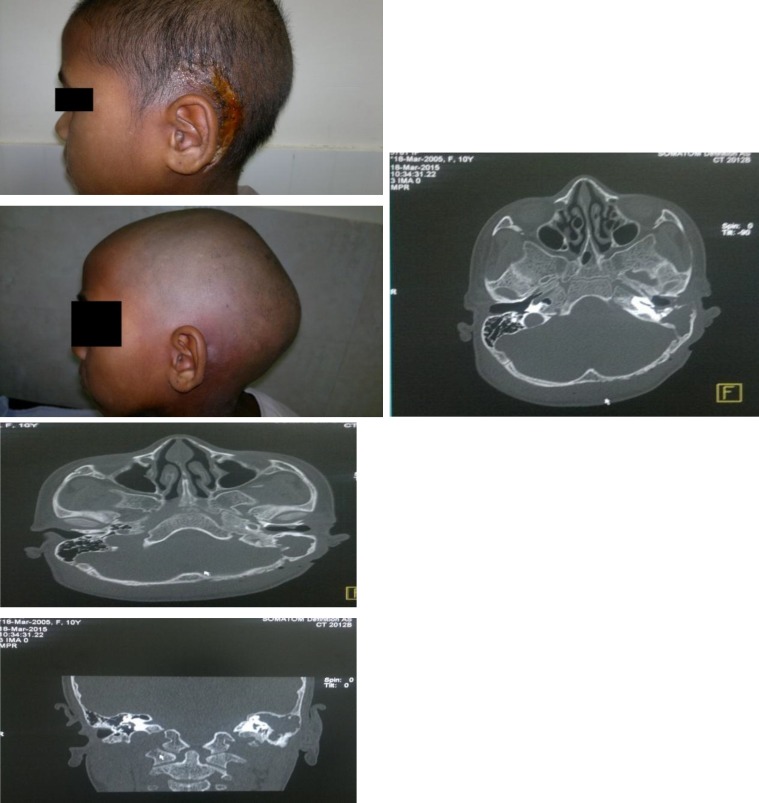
High resolution computed tomography of the temporal bone of the patient showing a soft tissue mass in the mastoid antrum, middle ear, left temporal and occipital area with erosion of the mastoid tip
